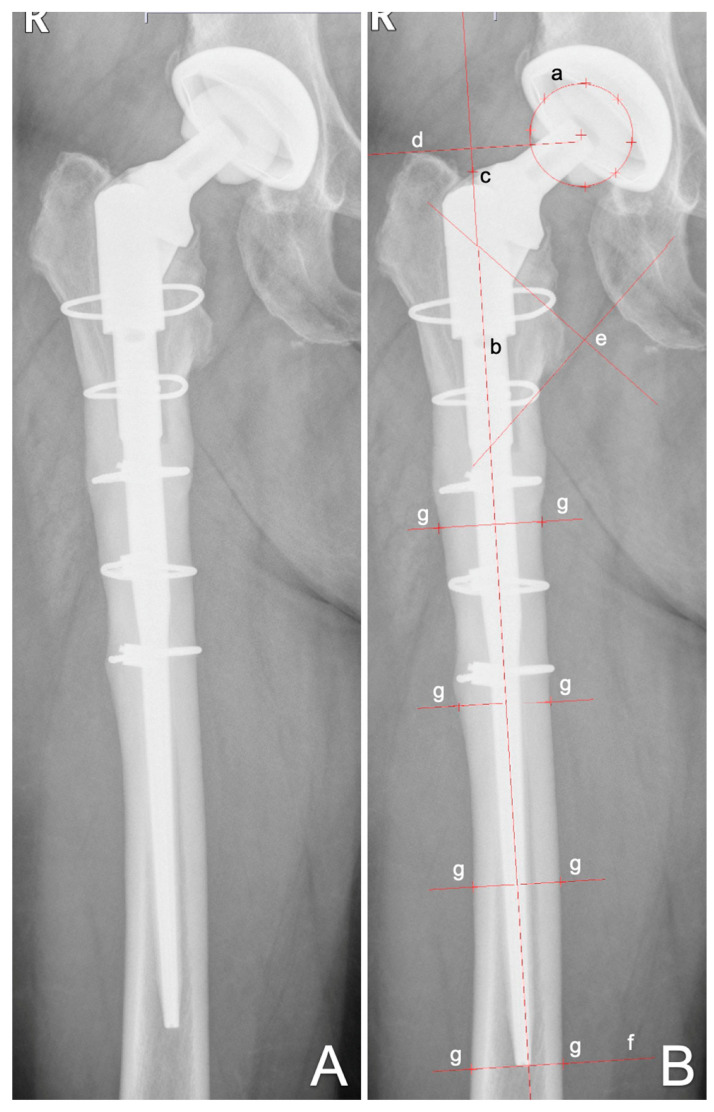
Anterior to posterior X-rays showing a cementless MP stem (A) and with EBRA-FCA references (B): (a) head points; (b) stem axis; (c) stem shoulder; (d) major trochanter line; (e) minor trochanter lines; (f) tip-of-stem line; (g) points at femoral bone contour.
